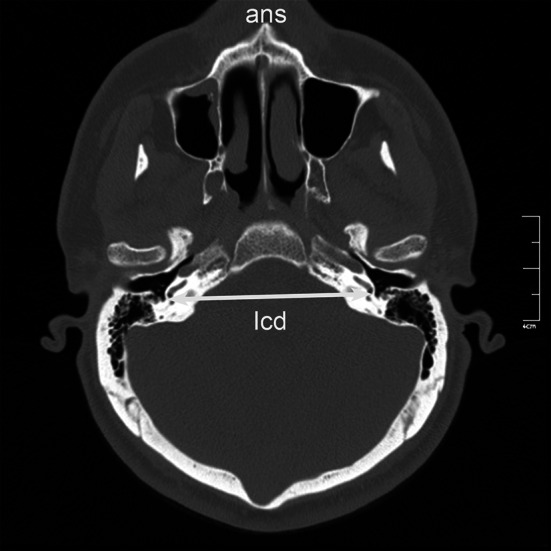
Reformatted computed tomography image showing intercochlear distance (*IcD*) and anterior nasal spine (*ans*)
